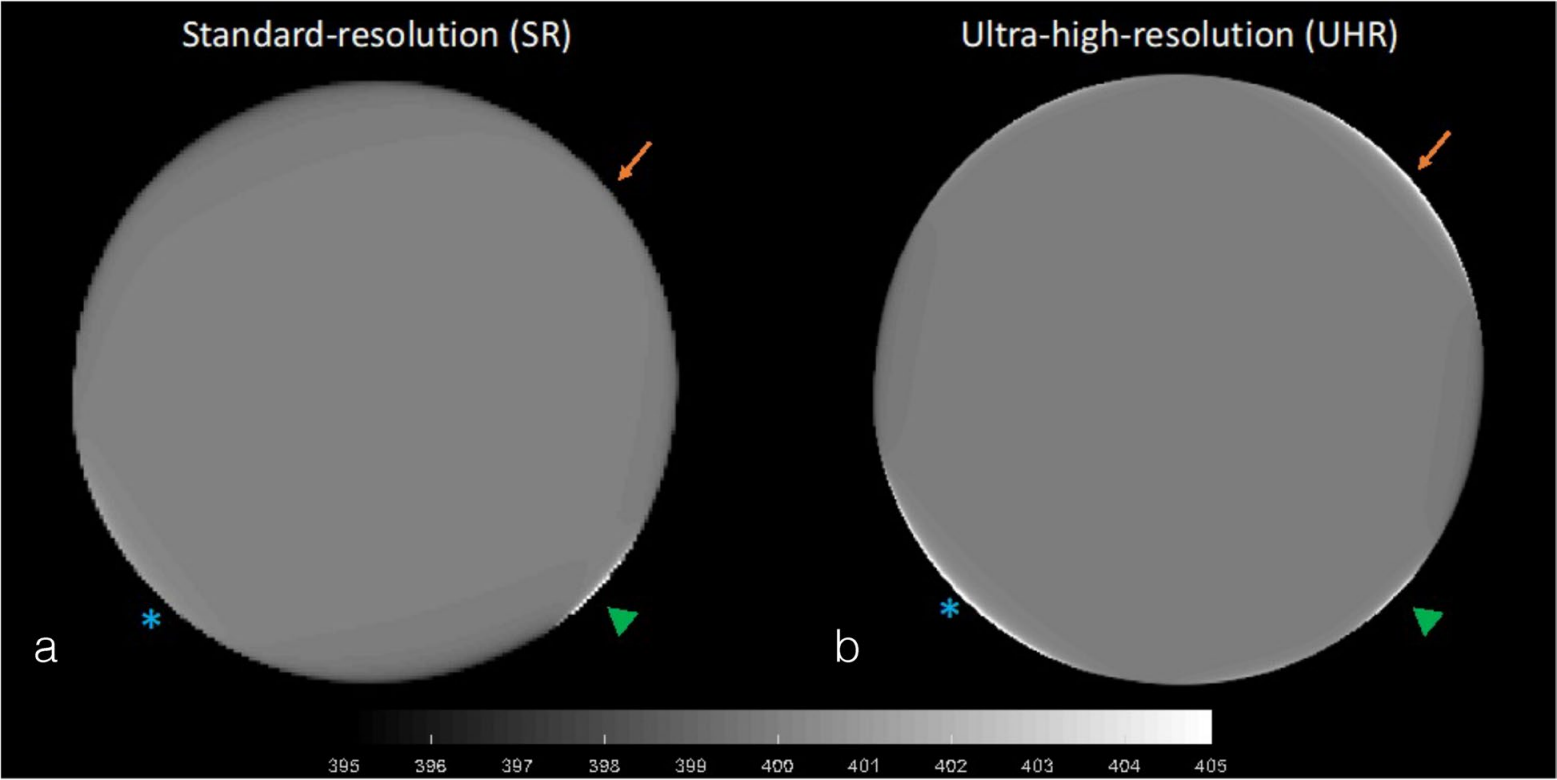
Sample CT images of a 3-cm diameter pulsating disk imaged at standard-resolution (a) and ultra-high-resolution (b), contaminated by pancreatic motion artifact. The diameter of the disk pulsated at the same rate and amplitude as the anterior–posterior displacement of the head of the pancreas measured by DENSE-MRI in subject #3. Cardiac-induced motion artifacts are more pronounced in the UHR image. The arrows, stars, and triangles mark some of the significant artifacts
